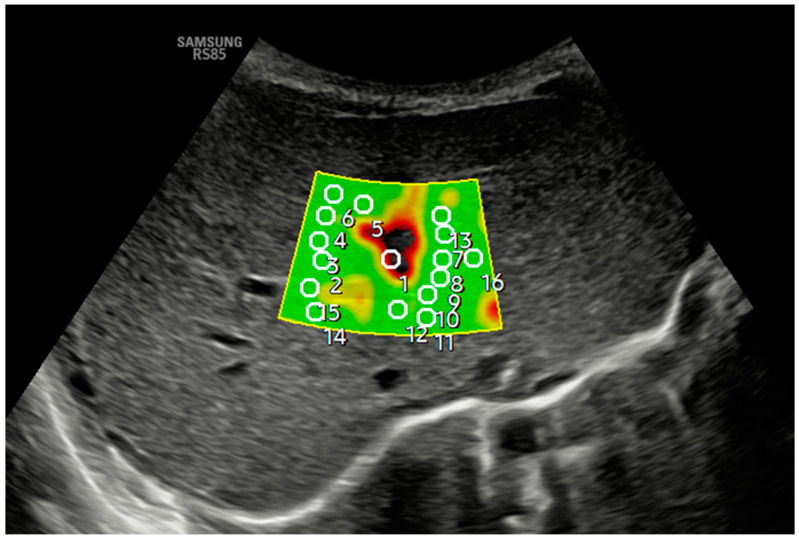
An axial section in segment IV obtained a B-mode ultrasound image in the liver anatomical plane, and samples were acquired with 2D-SWE using a convex probe. The measurement area is positioned, and the shear wavefront color map is generated. The color map, or elastogram, can be adjusted according to the color code assigned by each commercial house from highest to lowest stiffness or, as in the example, according to the quality of the measurement (in the example, green is optimal or very optimal measure quality), always selecting the one with the fewest artifacts and using the lowest possible ROI size. At least 10–15 valid measurements are obtained within the elastogram using the same validity criteria mentioned in the main text for pSWE. In the example, ROI 1 is invalid.
